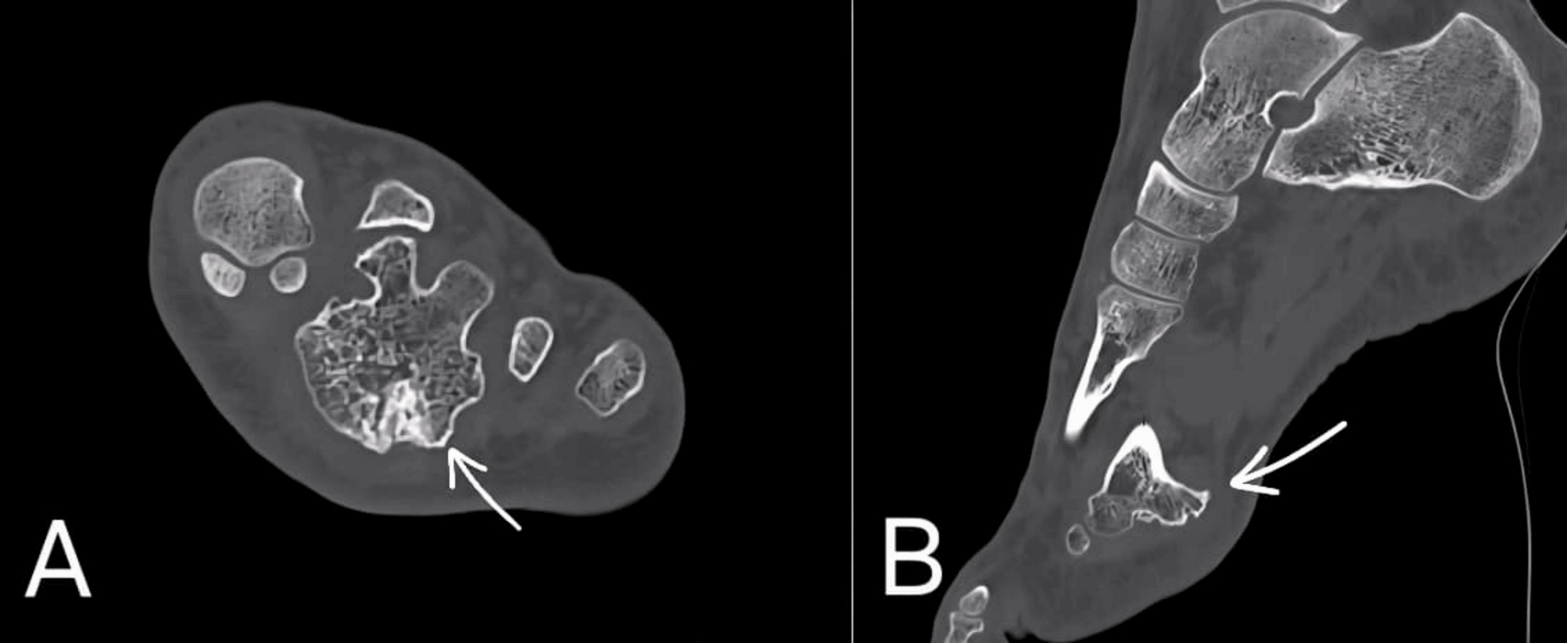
Coronal (A) and sagittal (B) CT images of the left foot showing a plantar exostosis arising from the distal third metatarsal (arrow), in close relation to the second metatarsal.
